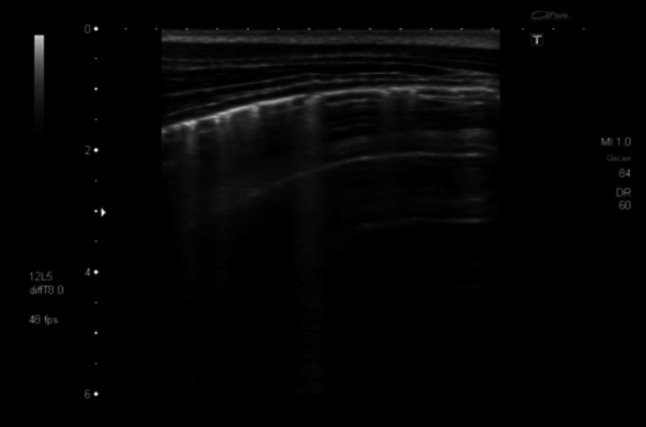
Transthoracic ultrasonography of the pleura. Multiple B-lines are visible suggesting an interstitial pattern of lung injury; as these are not penetrating the complete image, nor do they extinguish the A-lines completely which are visible towards the right side of the screen, these should be referred to as “lung rockets”
